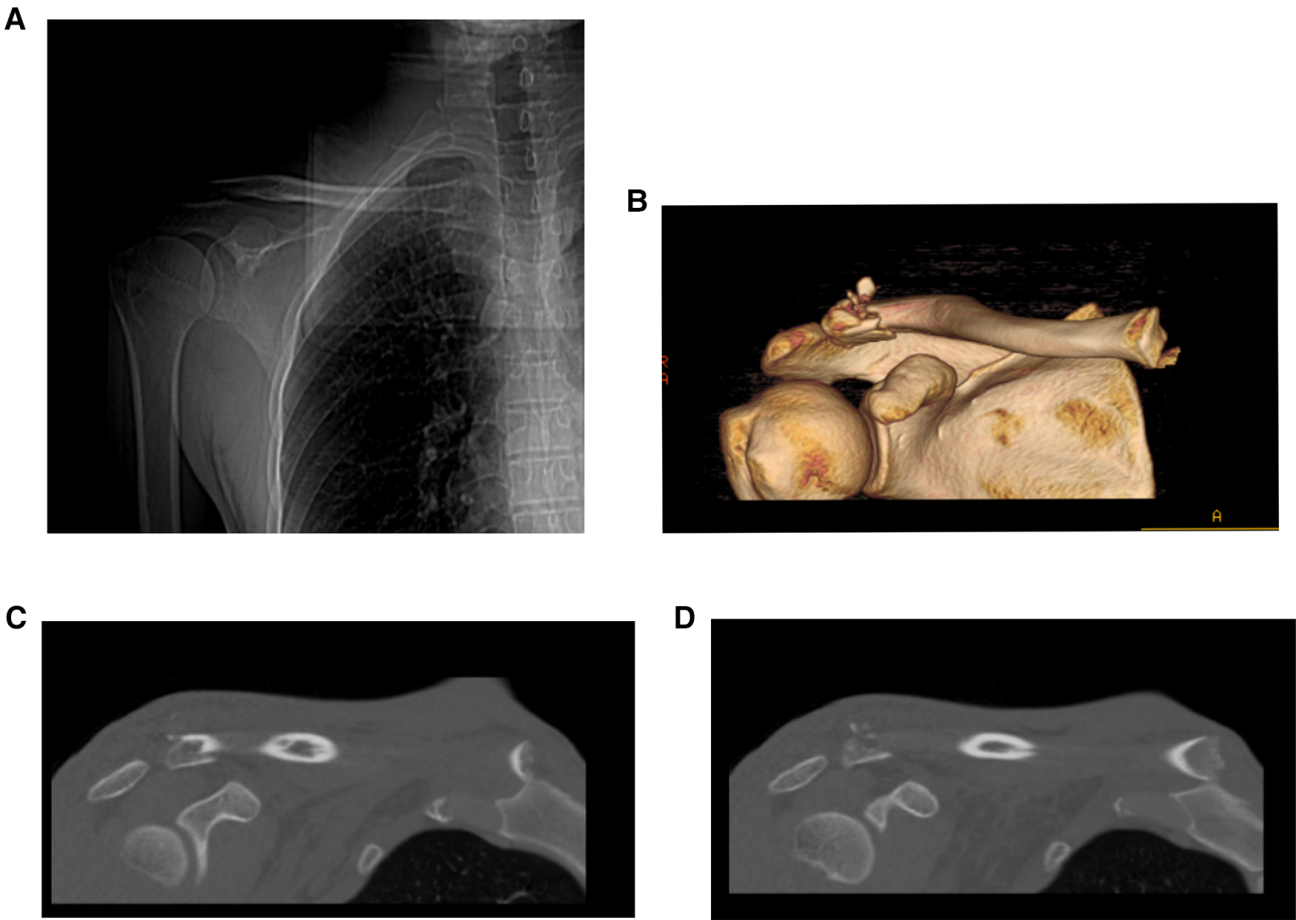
Imaging of a patient with a distal clavicle fracture with proximal clavicle dislocation. (A) X-ray images. (B–D) CT images.
